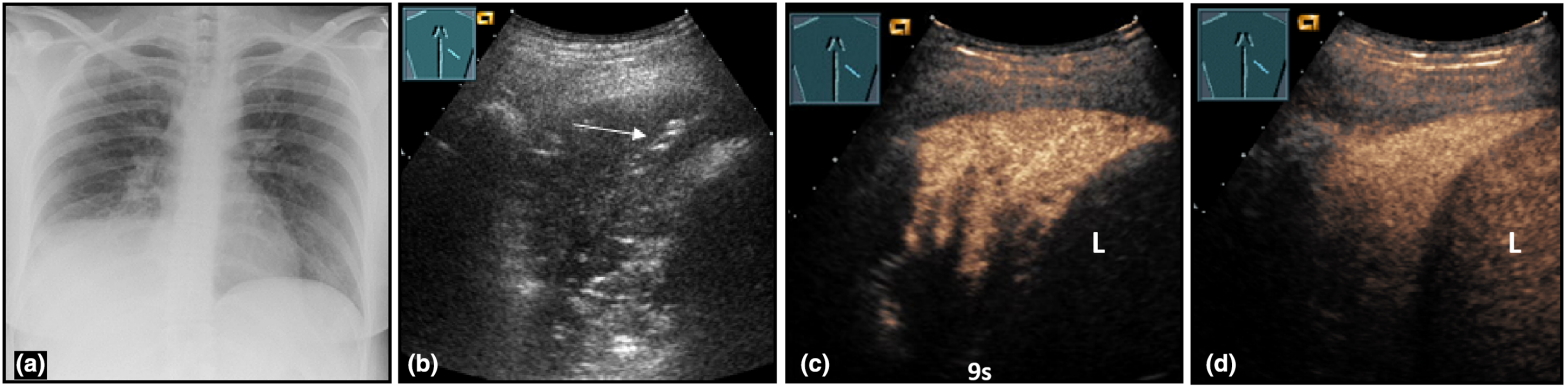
A 36‐year‐old female patient presented to the emergency department with cough, sputum, fever, and tachycardia. Visualisation of a right‐sided, low‐field consolidation on chest X‐ray (courtesy of Prof. Dr. Andreas H. Mahnken, Department of Radiology, University Hospital Marburg) (a); B‐mode ultrasound (b) shows a hypoechoic consolidation with air bronchogram (arrow); on contrast‐enhanced ultrasound, compared with the liver (L), after 9 s (c) the lesion shows a pulmonary arterial perfusion pattern, with a marked homogeneous enhancement after 2 min (d), consistent with community‐acquired pneumonia.
